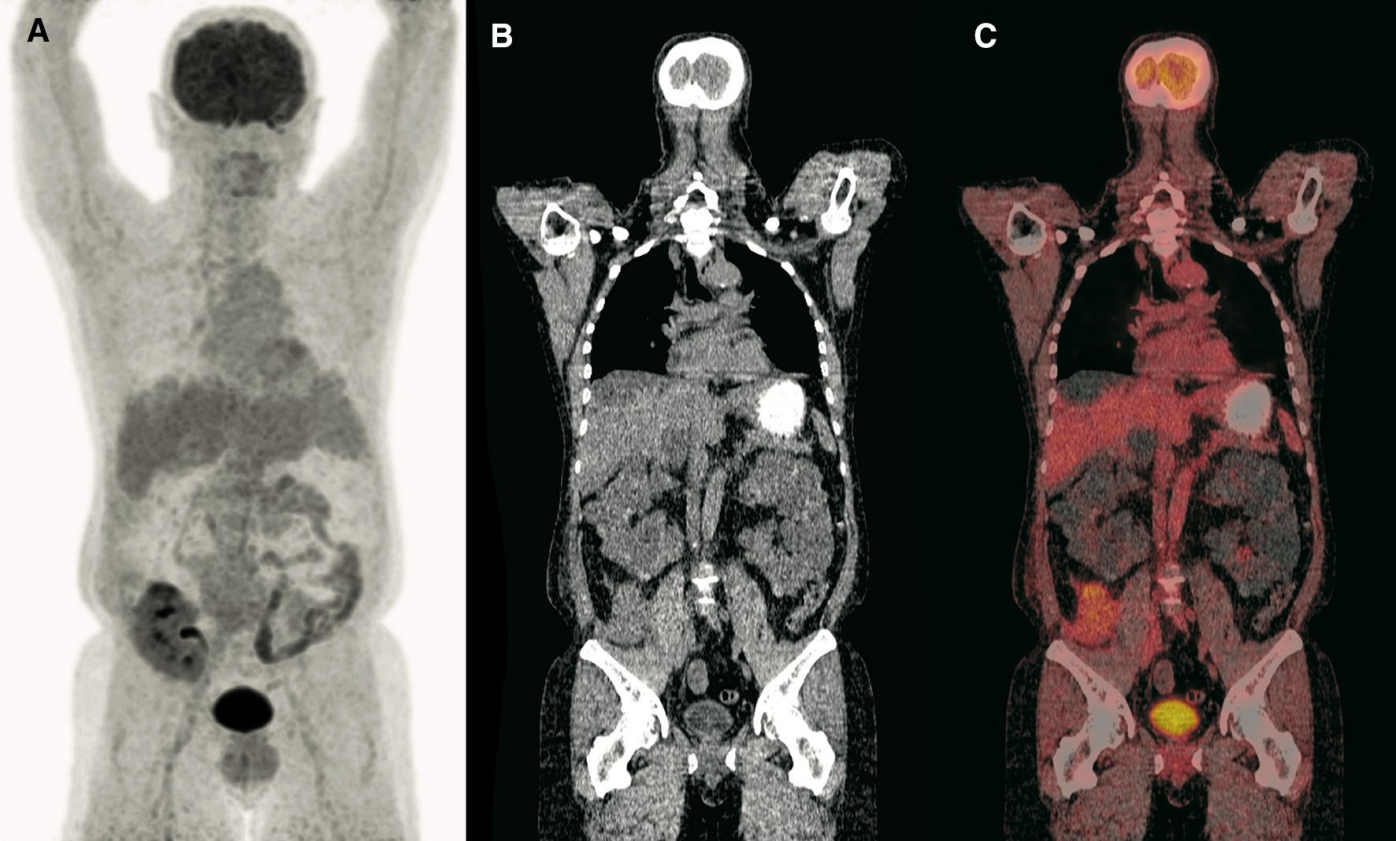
A 53-year-old man with ADPKD presented at the emergency department because of abdominal pain, fever and a possible urinary tract infection. He had received a kidney transplant 1 month before because of end-stage renal disease. Blood tests showed a CRP level of 19 mg/L and a white blood cell count of 8.6 × 10^9^/L, while a urine culture revealed Gram-negative rods. The patient was admitted, and an FDG-PET/CT scan was ordered to assess the native kidneys for infection. Coronal maximum intensity projection FDG-PET (a), low-dose CT (b), and fused FDG-PET/CT (c) did not show any signs of (cyst) infection in the native kidneys, and no pathologic FDG-avid foci elsewhere. FDG-PET/CT findings provided confidence to the clinicians to attribute the clinical and laboratory findings to a simple cystitis. The patient was sent home in good clinical condition with a course of nitrofurantoin for 3 days
